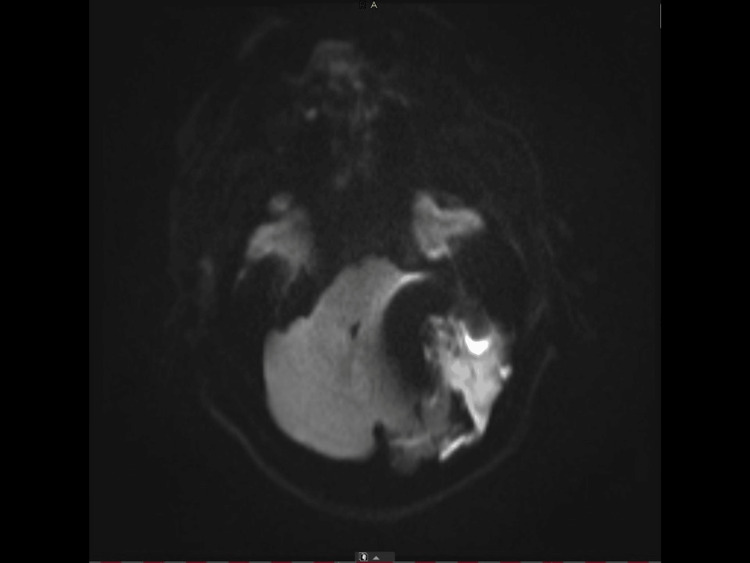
Preoperative MRI-DWI sequence showing diffusion restriction of the lesion content that was highly suggestive of an epidermoid cyst. MRI-DWI: Magnetic resonance imaging-Diffusion-weighted imaging
